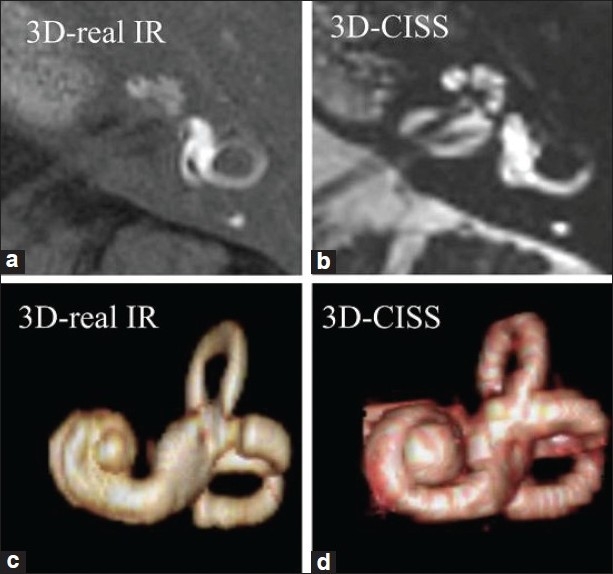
MRI protocols in a patient with Meniere’s disease. High spatial resolution 3D-real inversion recovery (IR) image (a, 0.8 mm thick) and 3D-constructive interference in the steady-state (CISS) image (b, 0.4 mm thick), and their volume-rendered (VR) images (c, d). By comparing the perilymphatic VR image (c) and total lymphatic VR image (d), we can appreciate the degree of endolymphatic hydrops three dimensionally (Naganawa S, Nakashima T. Cutting edge of inner ear MRI. Acta Otolaryngol 2009;129:15-21)
